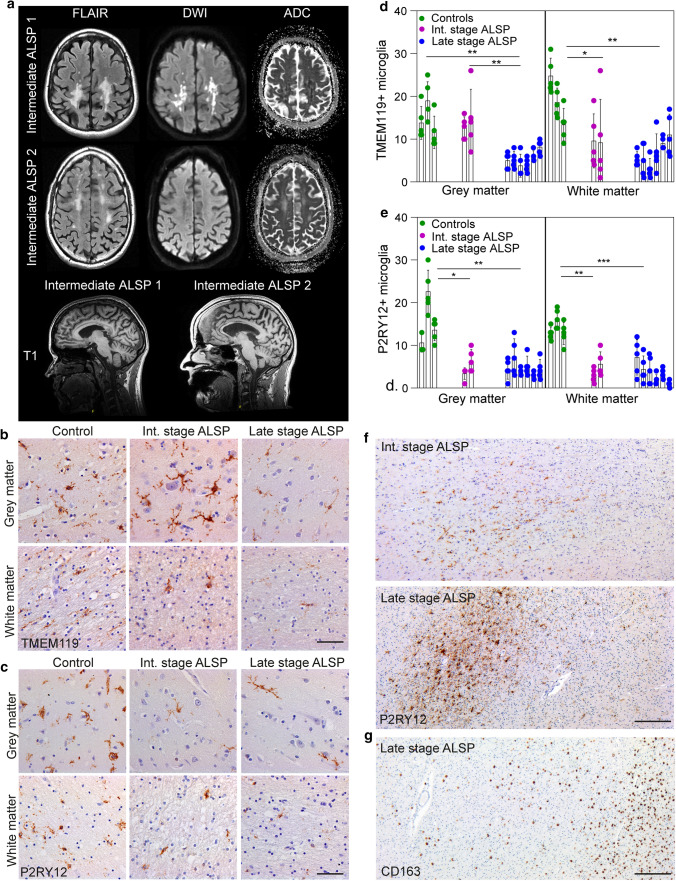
ALSP patients show an overall loss of (homeostatic) microglia and altered microglial distribution. a Brain MRI abnormalities in intermediate-stage ALSP patient 1 and intermediate-stage ALSP patient 2. Axial FLAIR images show hyperintense white matter abnormalities, most pronounced in the bilateral parietal and occipital lobes of intermediate-stage ALSP patient 1 and in the bilateral parietal and frontal lobes of intermediate-stage ALSP patient. Hyperintense signals on diffusion-weighted imaging (DWI) and corresponding low signal on apparent diffusion coefficient (ADC)-maps indicate restricted diffusion in the majority of the lesions in patient 1, but not in patient 2. Sagittal T1-weighted images show corpus callosum involvement and mild generalized brain atrophy in both patients. b Representative TMEM119 IHC images of the frontal gyrus of controls (*n* = 3), intermediate-stage ALSP patients (*n* = 2) and late-stage ALSP patients (*n* = 6) in grey matter (top row) and white matter (bottom row). c Representative P2RY12 IHC images of the frontal gyrus of controls (*n* = 3), intermediate-stage ALSP patients (*n* = 2) and late-stage ALSP patients (*n* = 6) in grey (top row) and white matter (bottom row). d, e Quantification of TMEM119 + (d) and P2RY12 + (e) microglia in grey (left) and white matter (right) of frontal gyrus. Data points represent microglia in one randomly taken image, 5 images/individual. f Representative P2RY12 IHC images of the frontal gyrus showing clustered distribution of P2RY12 + microglia in the white matter. g Representative CD163 IHC images of the frontal gyrus of late-stage ALSP (*n* = 6) patients showing clustered distribution of CD163 + cells in the white matter. Int.: intermediate. A nested one-way ANOVA test was preformed to test for significance (*p* < 0.05). Error bars represent SD. p* < 0.05, *p* < 0.01, p* < 0.001. Scale bars equal 50 μm (b, c) and 500 μm (f, g)
